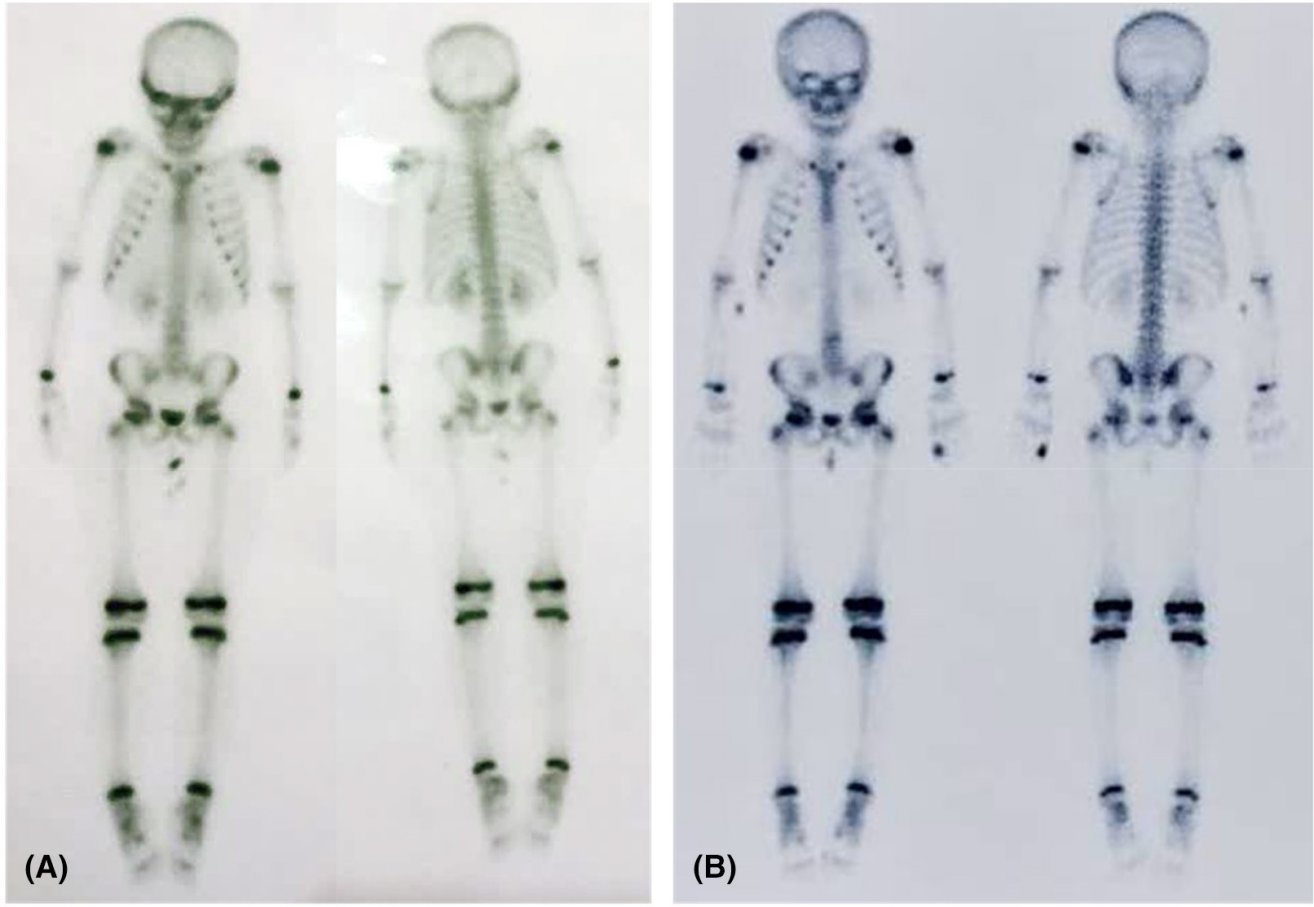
^99m^ Tc‐MDP skeletal scintigraphy; (A) before treatment. (B) seven months later, focal intense osteoblastic activity in the distal phalanx of the left‐hand second digit was seen.
